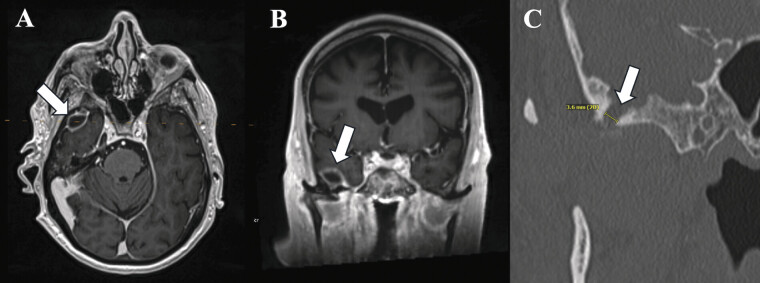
Case 3 postcontrast T1-weighted MR images in axial (
